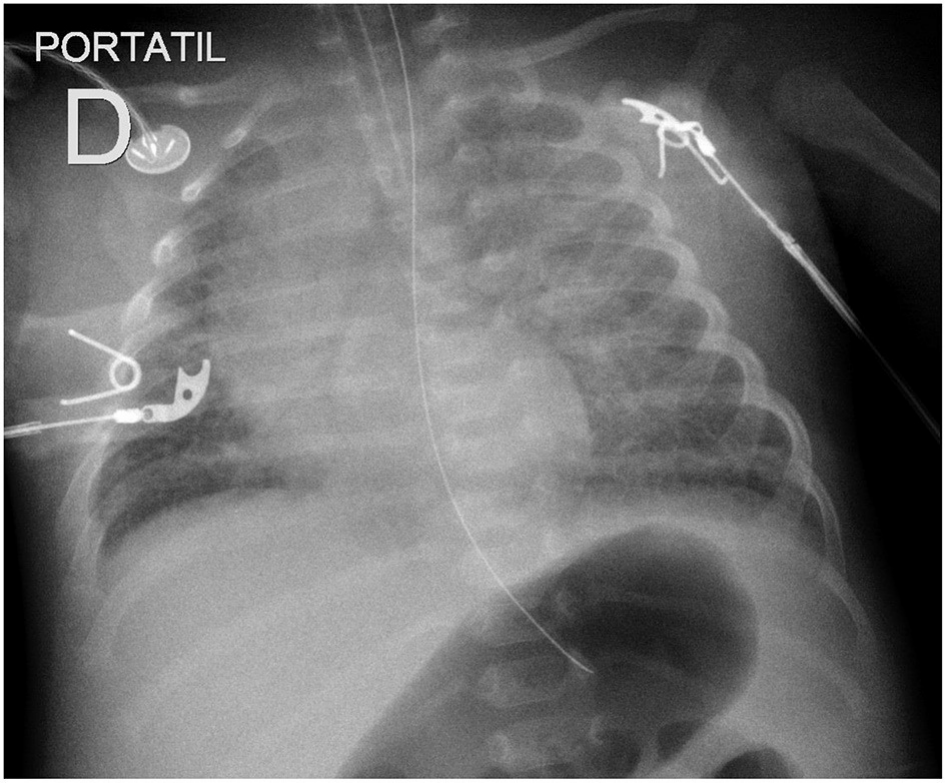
Chest X-ray showing diffuse interstitial and alveolar infiltrates in a newborn with Hantavirus infection.
